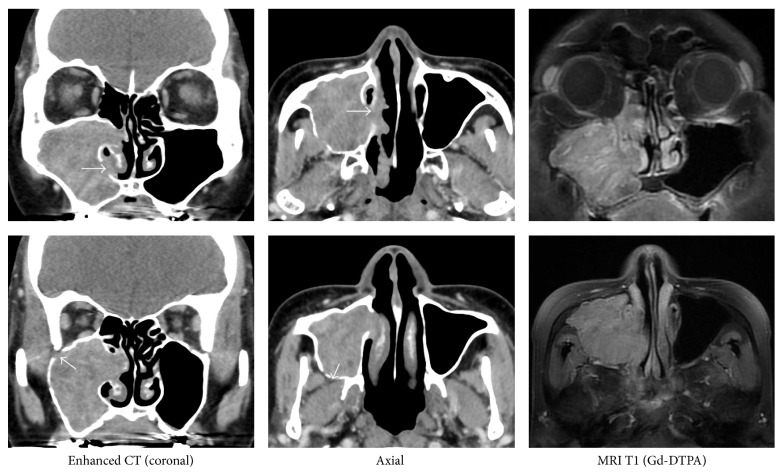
CT and MRI Imaging. The contrast CT shows bone defects in the anterior and medial walls of the maxillary sinus. There are no indications that the thickening of the bone should be suspected as the site of origin. MRI T1 (Gd-DTPA) shows secondary maxillary sinusitis and a serpentine cerebriform filamentous structure, but there is no mass to otherwise indicate a possible origin.
